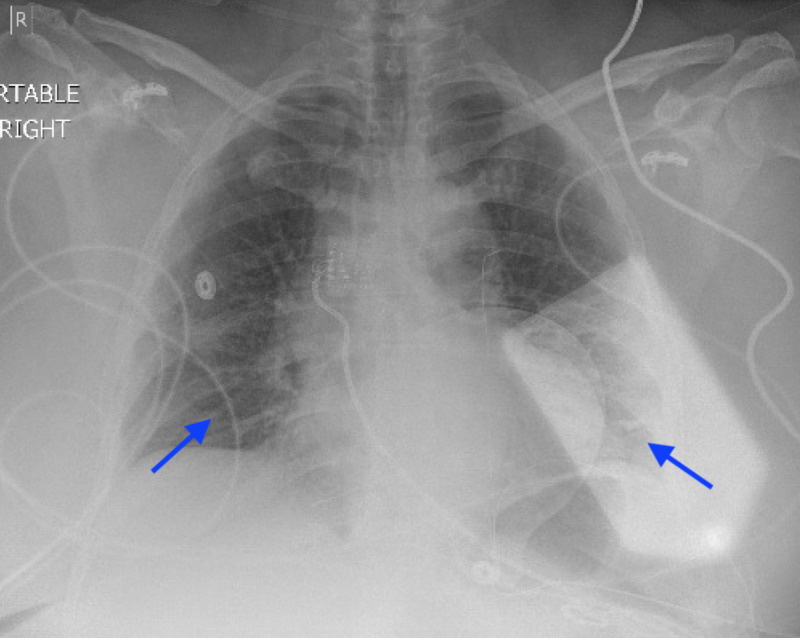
Portable chest X-ray Portable chest X-ray showing bilateral patchy airspace opacities.
